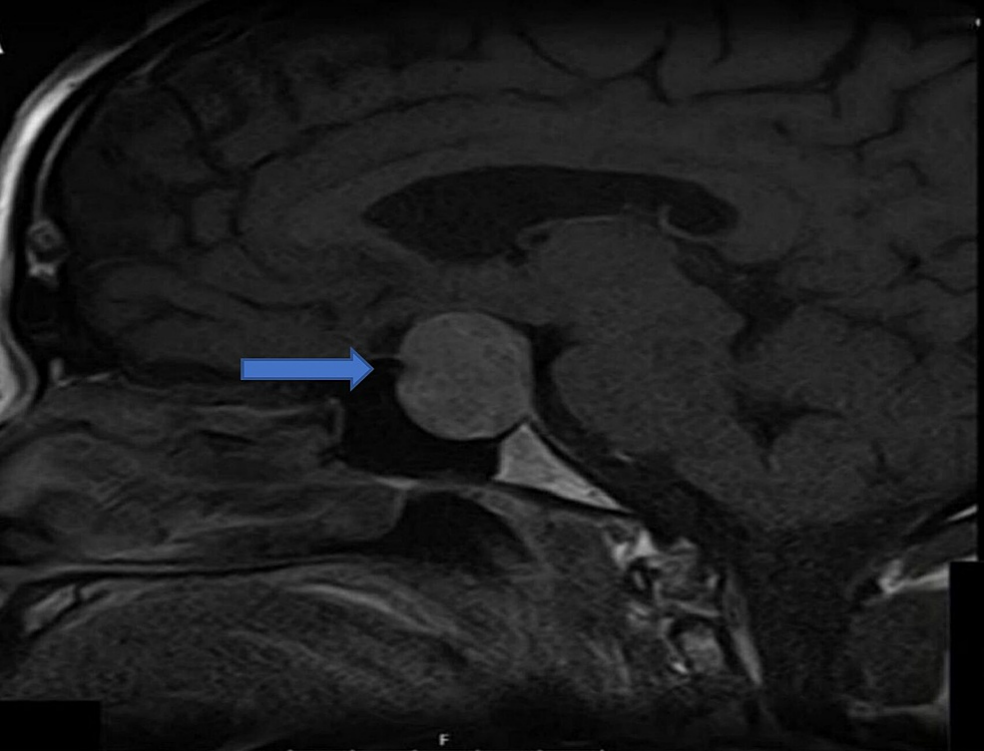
MRI head showing pituitary tumor
